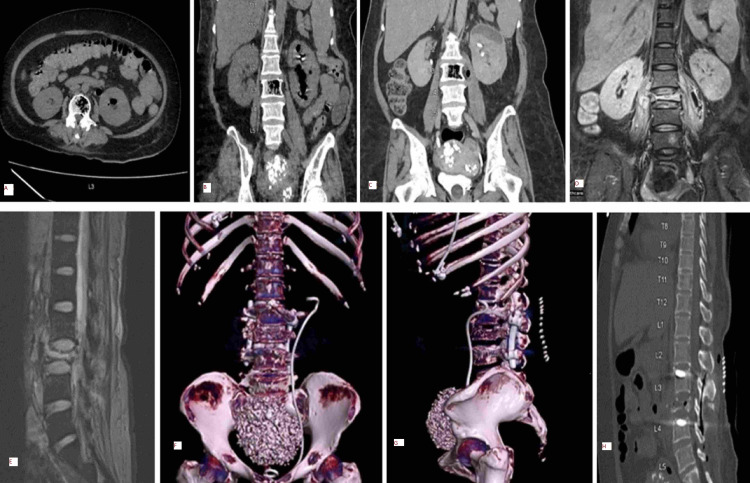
Imaging of the patient during her stay in hospital 1A: CT abdomen transverse plane shows air foci in left kidney and L3 vertebra 1B: CT abdomen coronal view shows air foci in left kidney, L3 vertebra, and enlarged left psoas muscle 1C: CT cut showing emphysematous osteomyelitis with extension to the left psoas abscess 1D: MRI during the second admission shows complete L3 collapse with psoas abscess and pyelonephritis 1E: MRI shows the abscess extending from the destroyed vertebra, extradurally, along the thecal sac with compression of cauda equina nerve roots and narrowing of neural foramina at the L2-L3 level 1F, 1G: postoperative CT volume-rendered image shows the destroyed vertebra, with the double-J stent in the left kidney 1H: sagittal CT shows post-surgical debridement status CT: computed tomography; MRI: magnetic resonance imaging
